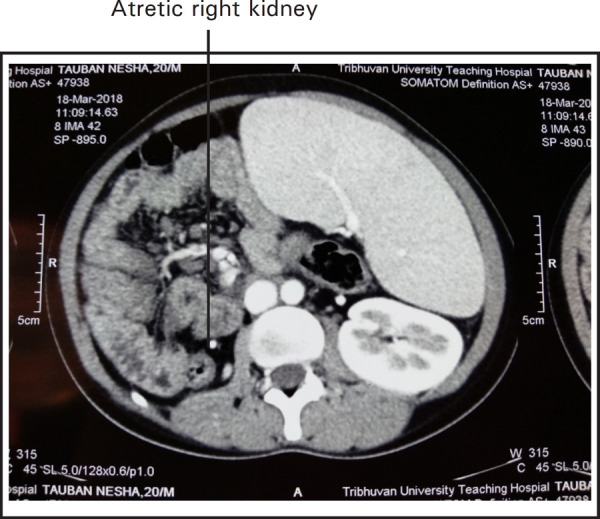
CT scan showing solitary left kidney with splenomegaly.
